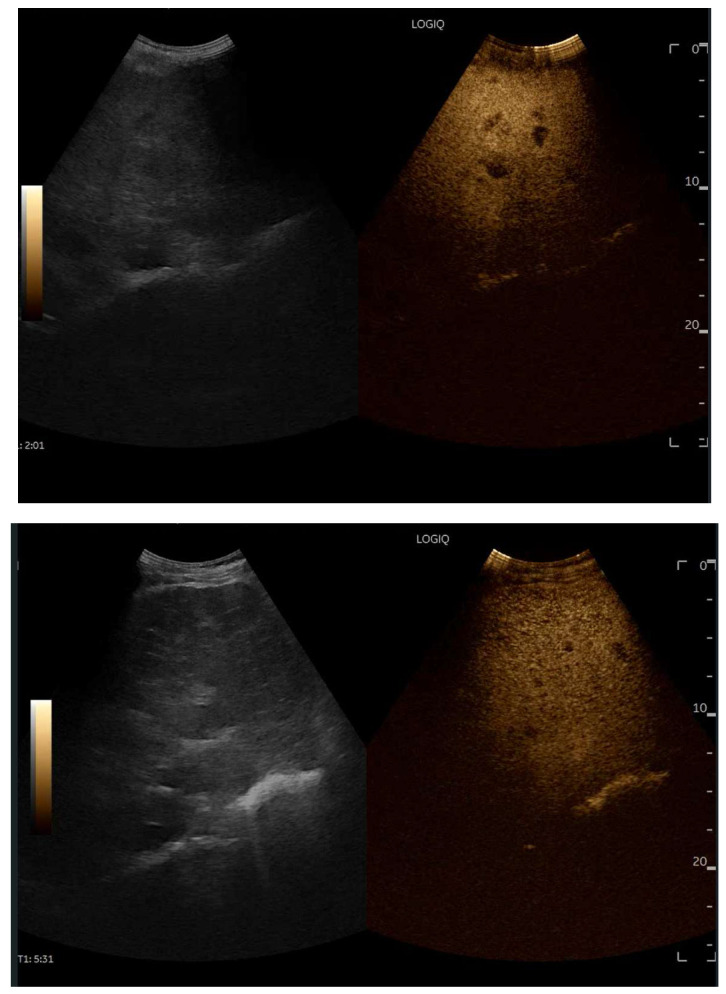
Contrast-enhanced ultrasound (CEUS) in the late phase shows the appearance of hypo-vascular areas due to varying degrees of congestion and hepatic alteration. These areas may mimic malignant nodular lesions with early washout.
